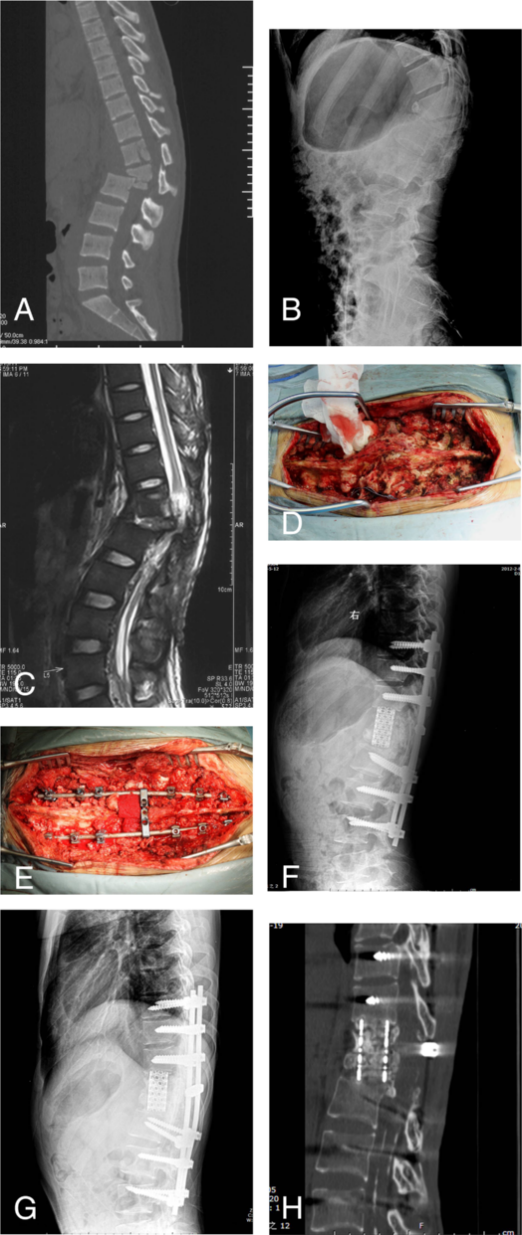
Radiographs and photographs of case 7. (A) Sagittal computed tomography scan of the initial injury showed dislocation at L1/L2 levels and significant comminution of the L1 vertebral body. (B) Preoperative lateral X-ray film: Collapse of the anterior column after implant removal and local kyphosis of 52°. (C) Preoperative MRI T2-weighted film. Intraoperative photographs of thoracolumbar spine before (D) and after (E) correction. (F) Immediately postoperative lateral radiograph: The kyphosis was corrected to 12°. (G) Lateral film at 2-year follow-up: Good maintenance of the correction and 4° of correction loss was noted. (H) Computed tomography scan at 2-year follow-up: Solid bony intervertebral fusion was achieved.
